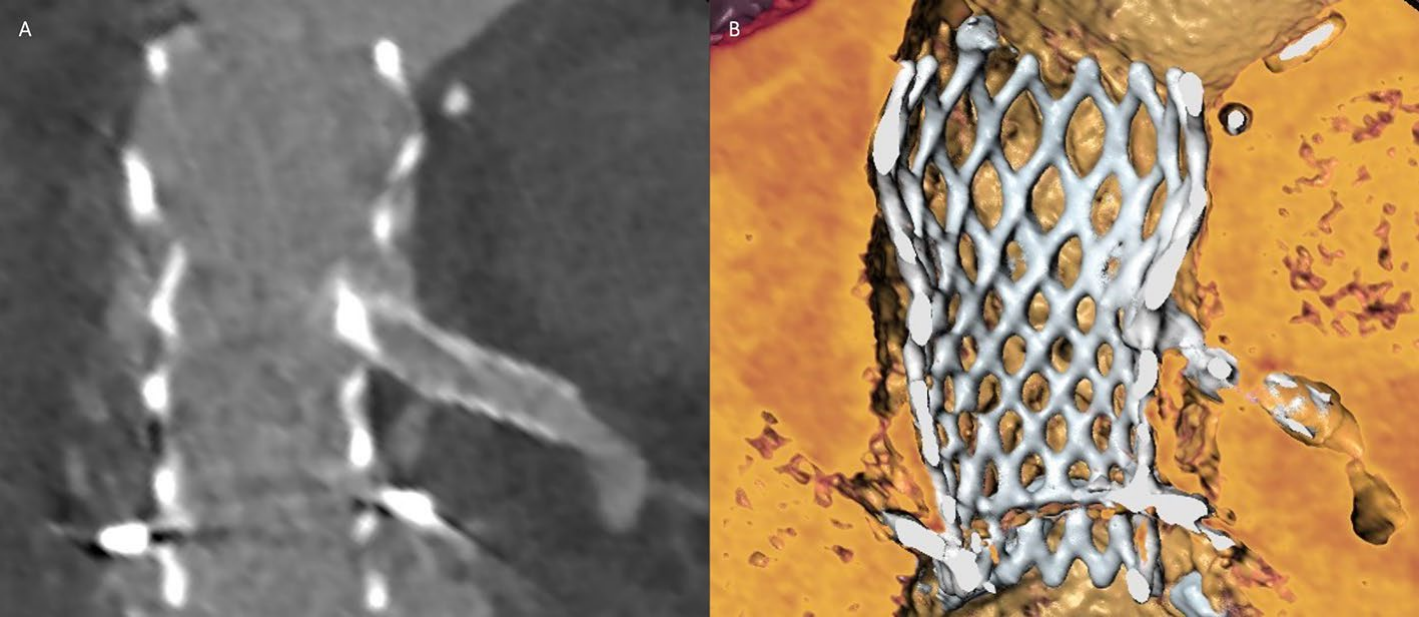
Chimney stenting. A Chimney stent implantation in left coronary artery after BASILICA failure (CoreValve Evolut R in Sorin Mitroflow), post-TAVI CT. B post-TAVI three-dimensional CT reconstruction (see A). *BASILICA* bioprosthetic or native aortic scallop intentional laceration to prevent iatrogenic coronary artery obstruction, *CT* computed tomography, *TAVI* transcatheter aortic valve implantation
